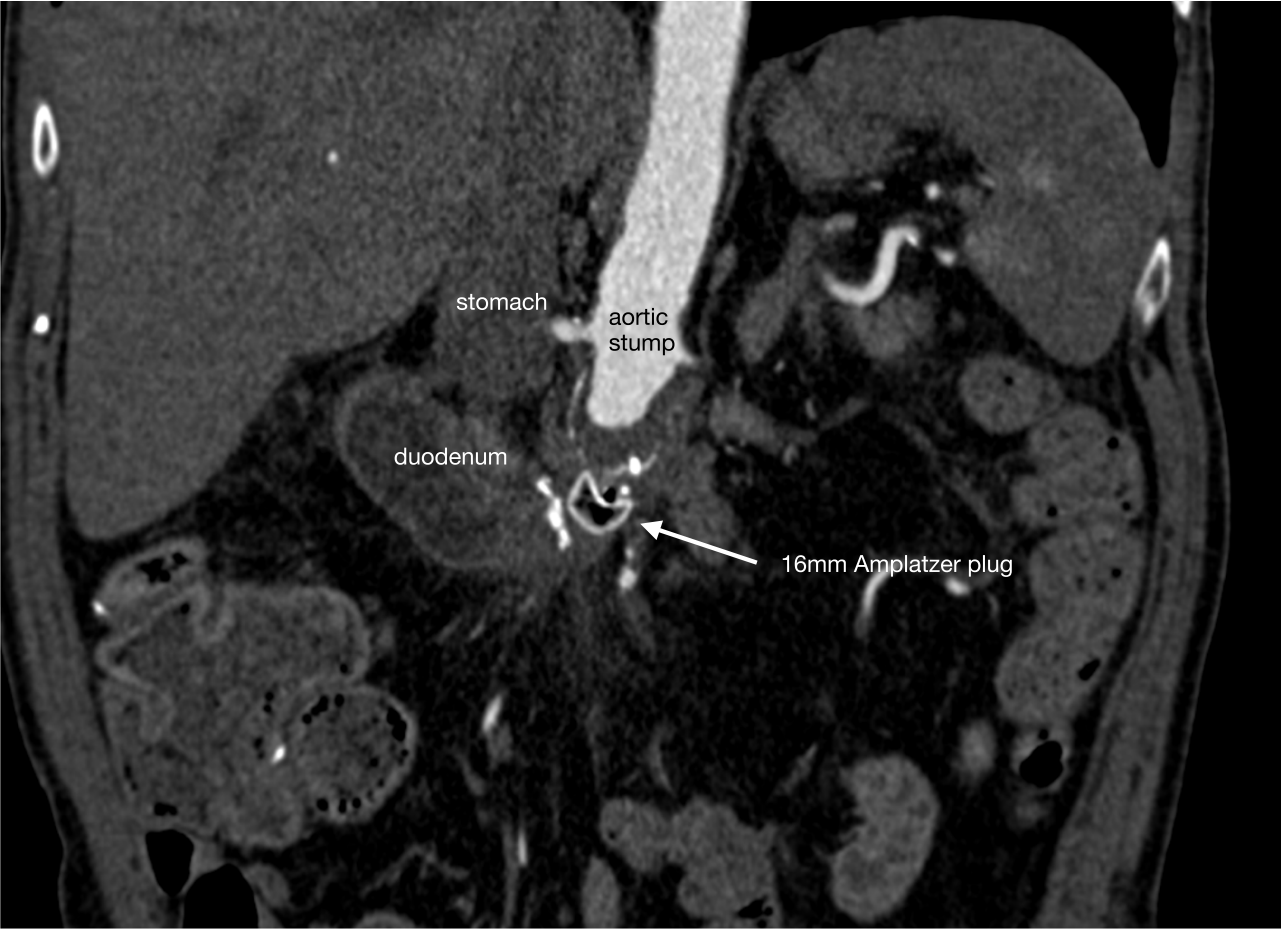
CTA abdomen with the Amplatzer® plug in situ after 3 months. CTA after 3 months showed thrombosis of the aortic stump with the Amplatzer® Vascular Plug in situ
